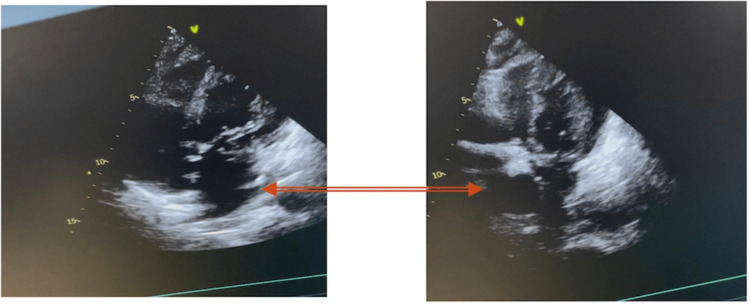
Presurgical echocardiography Images in systole and diastole show normal ejection fraction (55%), with no regional wall abnormalities or valvular defects.
